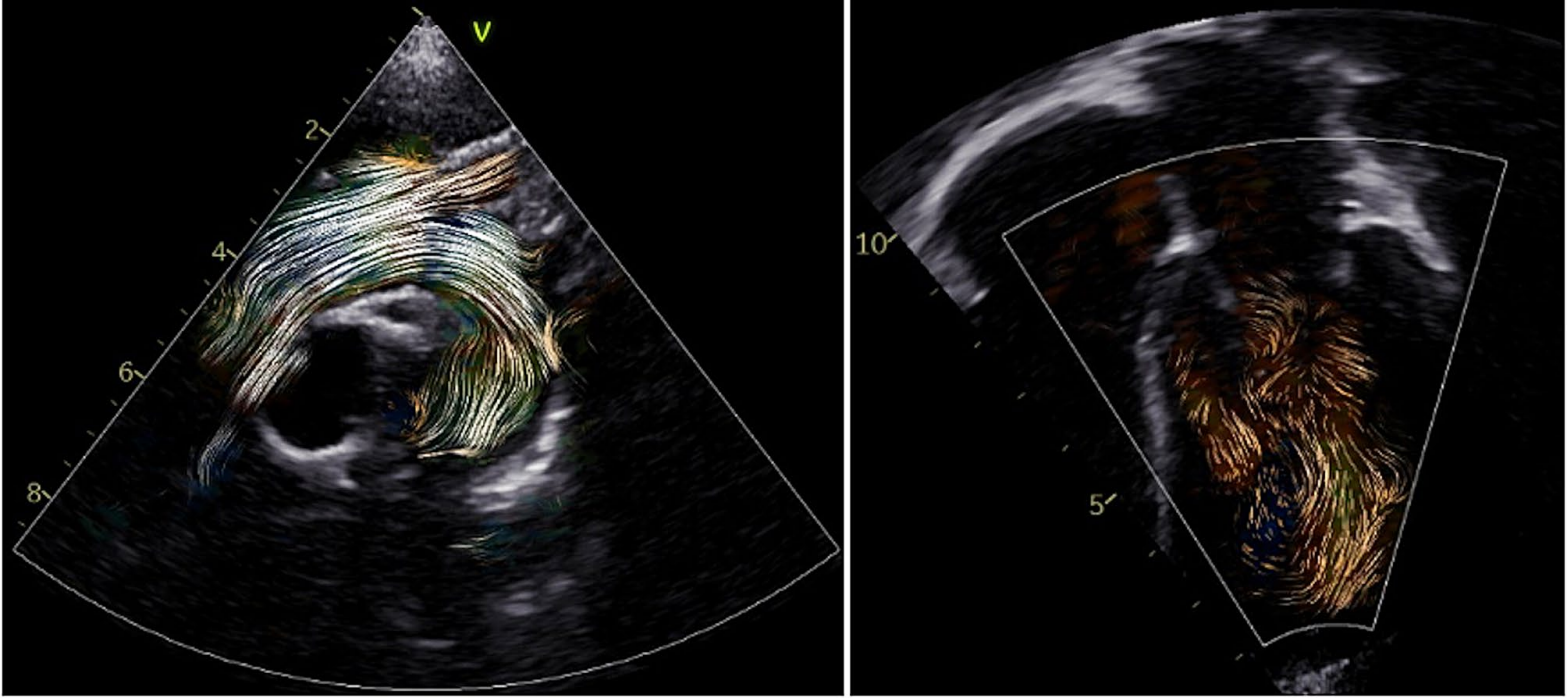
Intracardiac flow analysis using blood speckle tracking echocardiography. Fluid dynamic analysis using blood speckle tracking of the aortic arch (left) and the left ventricle (right) in a healthy child
